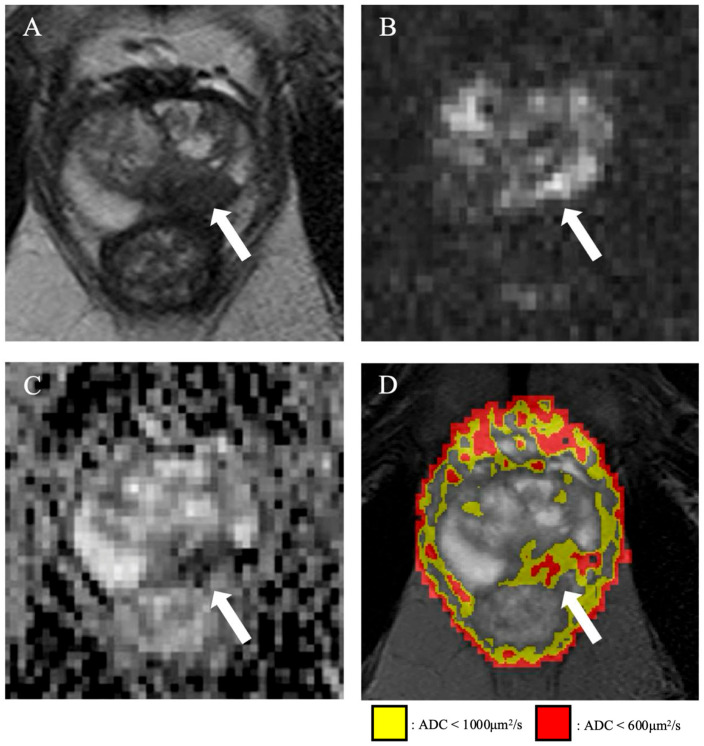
Bi-color map by the ADC value. (A) Hypointense lesion in the left peripheral zone on the T2-weighted image (arrow). (B) Hyperintense lesion on the high b-value diffusion-weighted image (arrow). (C) Hypointense lesion on the Apparent Diffusion Coefficient map (arrow). (D) The lesion with ADC < 1000 µm^2^/s and <600 µm^2^/s is presented in yellow and red, respectively. Lesions with ADC < 1000 µm^2^/s, including that with ADC < 600 µm^2^/s, are considered suspect lesions for clinically significant prostate cancer (arrow).
